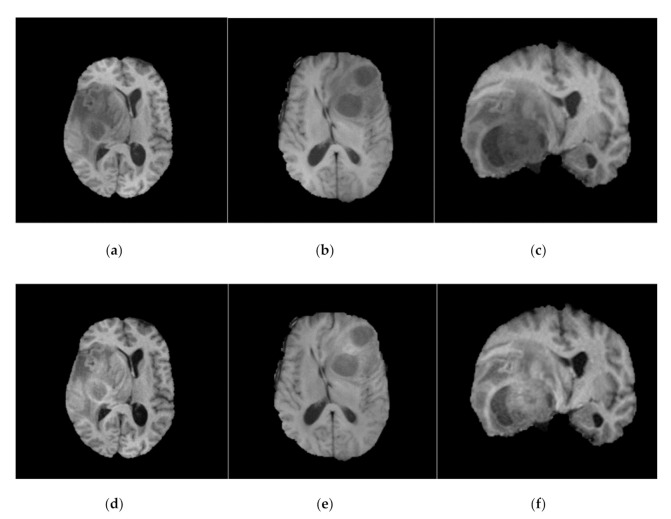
Before (top row) and after (bottom row) bias field correction. Each MR image in the top row (a–c) has the corresponding bias field corrected MR image (d–f) in bottom row. All the images shown here are FLAIR (for fair comparison).
